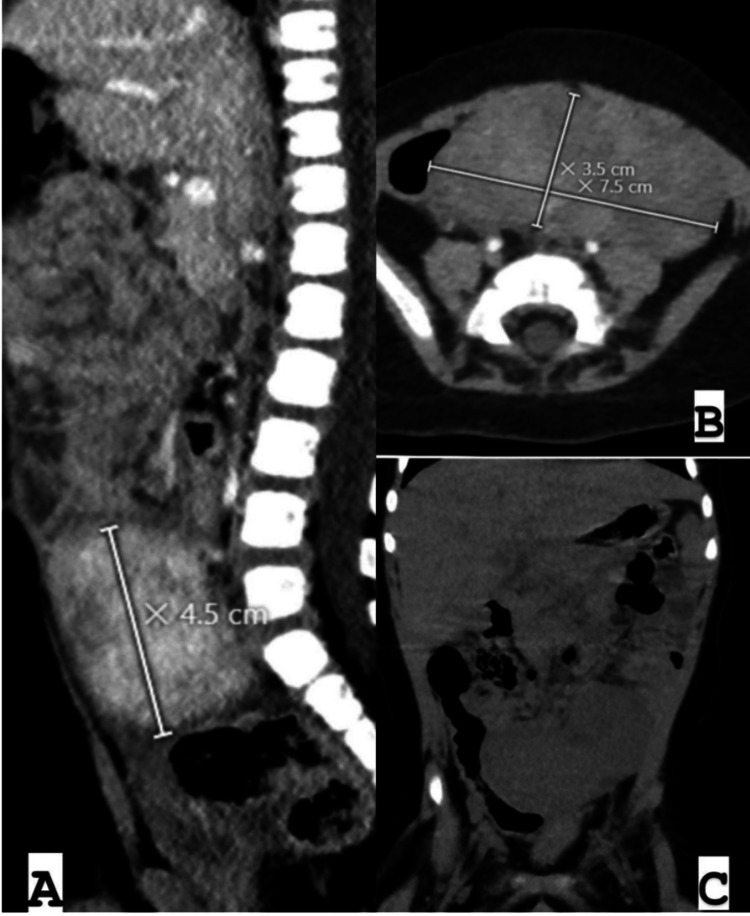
Abdominal CT scan CT scan showed a well-defined, bilobed, heterogeneously enhancing mass lesion measuring approximately 7.5 × 3.5 × 4.5 cm, with surrounding inflammatory changes and free fluid in the left paramidline lower abdomen, anterior to the iliac vessels, displacing adjacent bowel loops (A: sagittal view, B: axial view, C: coronal view).
